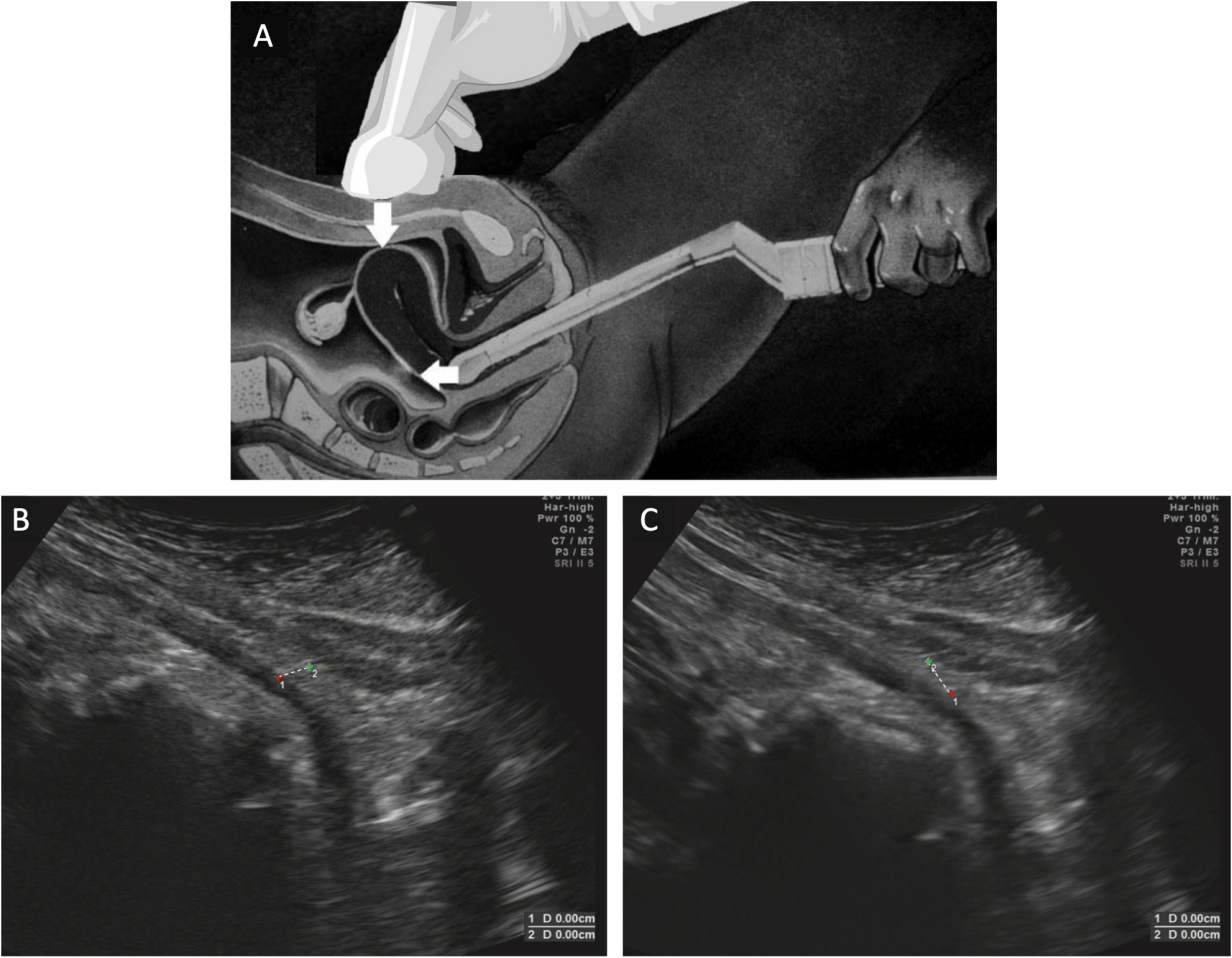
Sliding sign assessment using dynamic transvaginal sonography. (A) Transvaginal probe is inserted into the vaginal and then placed in the front wall of the vagina using gentle pressure and release motions in order to observe movement between the vesicouterine fold and the anterior uterine wall. Transabdominal probe is placed directly above perpendicular to the caesarean section incision mark. During examination, the probe was slightly moved back and forth along the scar and perpendicular to it. (B) The initial position before the patient was instructed to breathe; point 1 is located on the anterior uterine wall and point 2 is on the muscle fascia tissue. (C) The presence of uterine displacement against the abdominal wall was confirmed by a positive sliding sign. The position of point 1 on the anterior uterine wall has shifted downwards whereas point 2 on the muscular fascia has remained in its original position during the patient’s breathing. Modified with permission from Reid & Condous (2013) and Baron et al. (2018).
